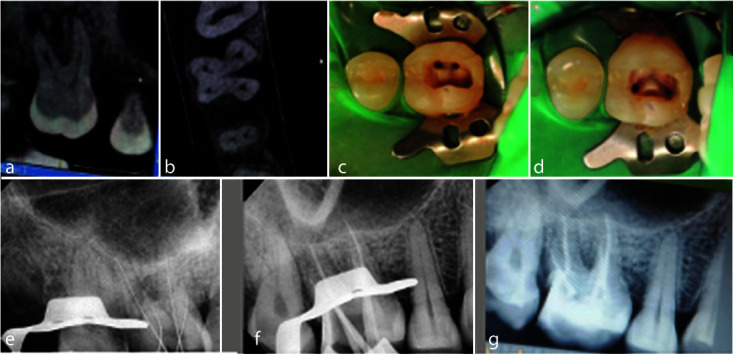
a-b: Pre-operative cone beam computed tomography (CBCT) images, c: Mesiobuccal and distobuccal canal orifices, d: Two
